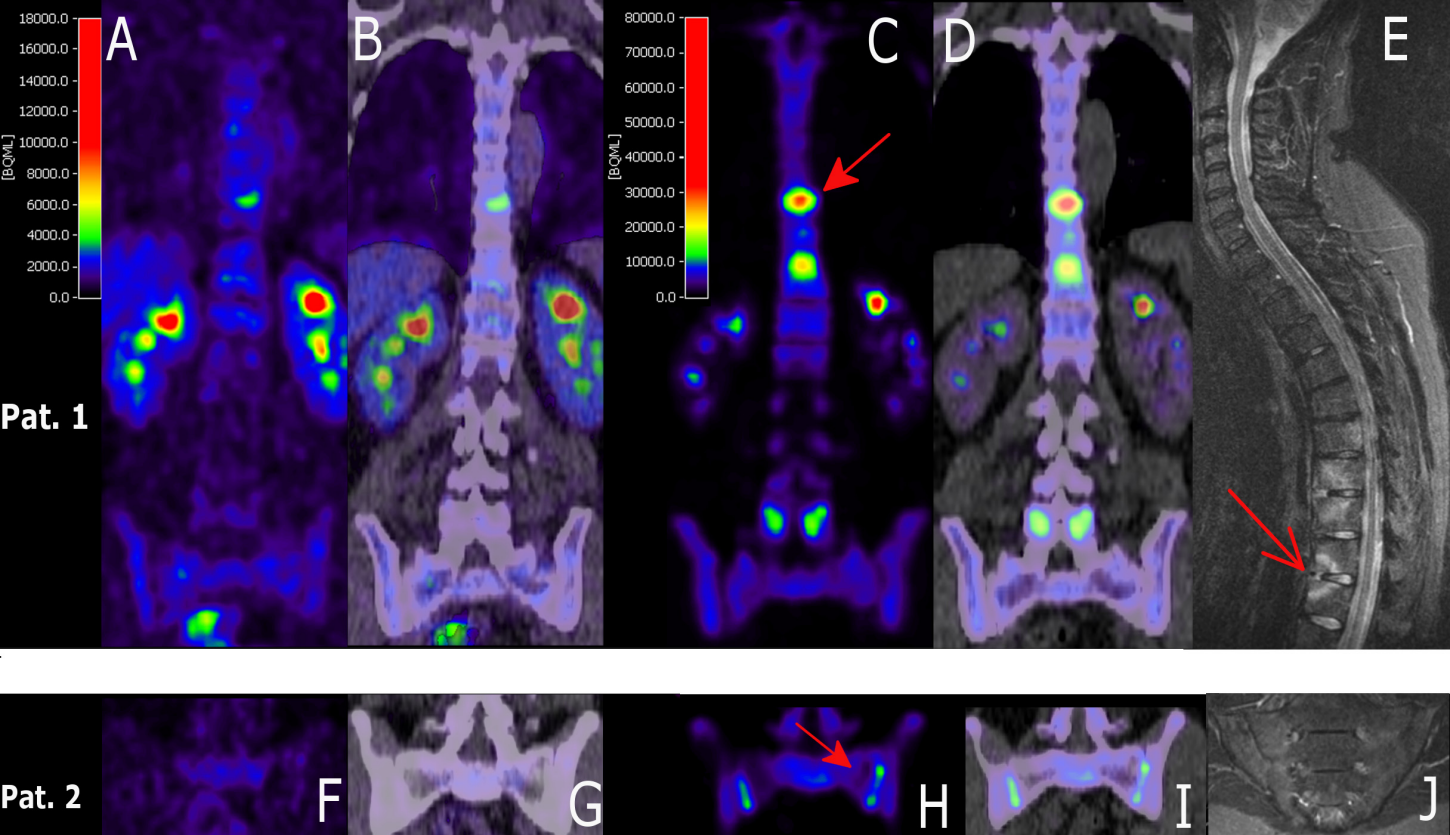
[^18^F]FDG and [^18^F]fluoride PET-CT and MRI images of patients 1 (a-e) and 2 (f-j). Both patients underwent an extra [^18^F]fluoride PET-CT scan. Coronal PET (a, c, f, h) and PET-CT (b, d, g, i) images obtained with [^18^F]FDG (a, b, f, g) and [^18^F]fluoride (c, d, h, i). Multiple hotspots are shown; two examples are indicated by the red closed arrows (c, h). (e) Sagittal MRI image (STIR) of vertebral column and (j) coronal/oblique MRI (STIR) of sacroiliac joints. Multiple lesions with increased signal (bone marrow edema) are shown; an example is indicated by the open arrow. [^18^F]FDG, [^18^F]-fluoro-2-deoxy-D-glucose; MRI, magnetic resonance imaging; PET, positron emission tomography; PET-CT, positron emission tomography-computed tomography; STIR, short-tau inversion recovery.
